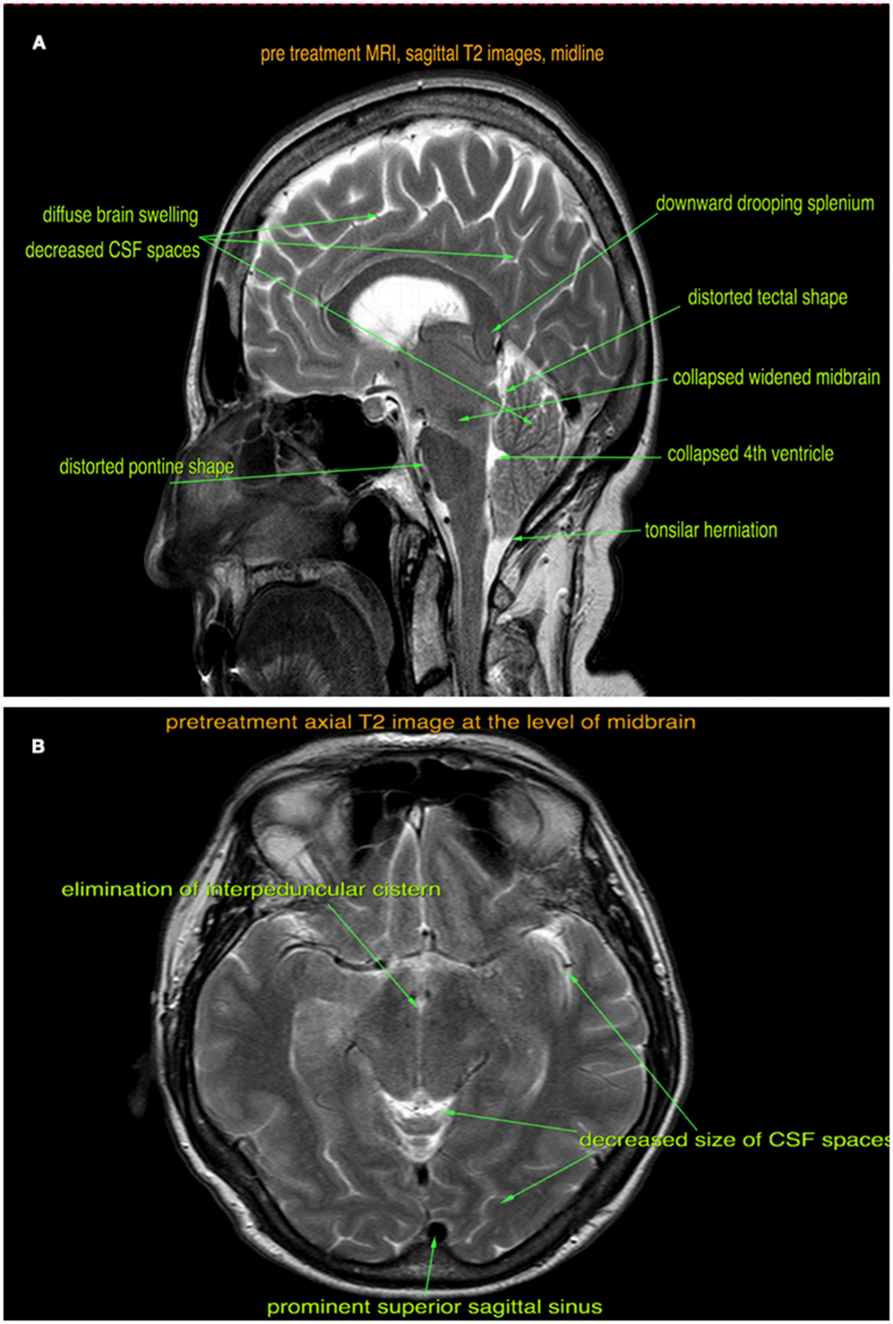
Pre-treatment sagittal (A) and axial (B) T2-weighted MRI images show brain sagging, tonsillar herniation, diffuse brain swelling, and venous sinus congestion.
